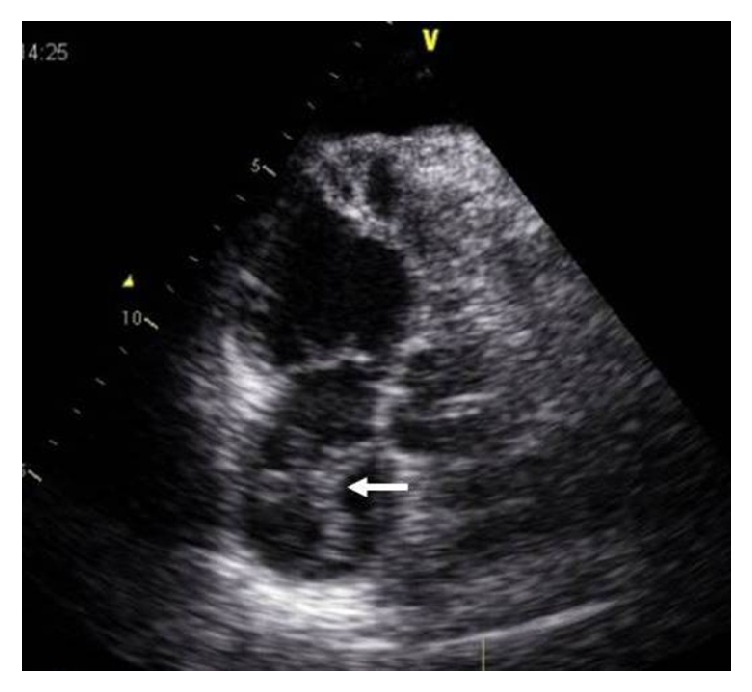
Transthoracic echocardiography apical view showing a mobile thrombus in the right atrium in systole (white arrow).
